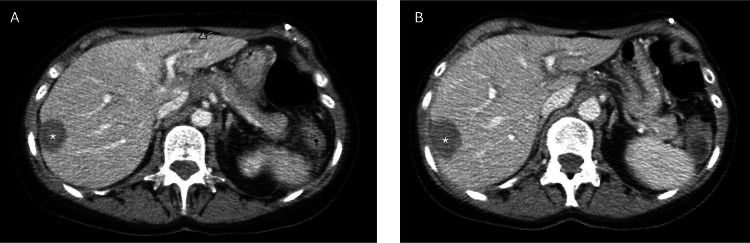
Resolution of liver metastasis after initiation of treatment with fam-trastuzumab-deruxtecan. CT A/P prior to treatment shows a sub-centimeter left liver lobe metastasis (A; arrow), now resolved after treatment with fam-trastuzumab-deruxtecan (B). Asterisk (*) indicates area of liver ablation treatment.
